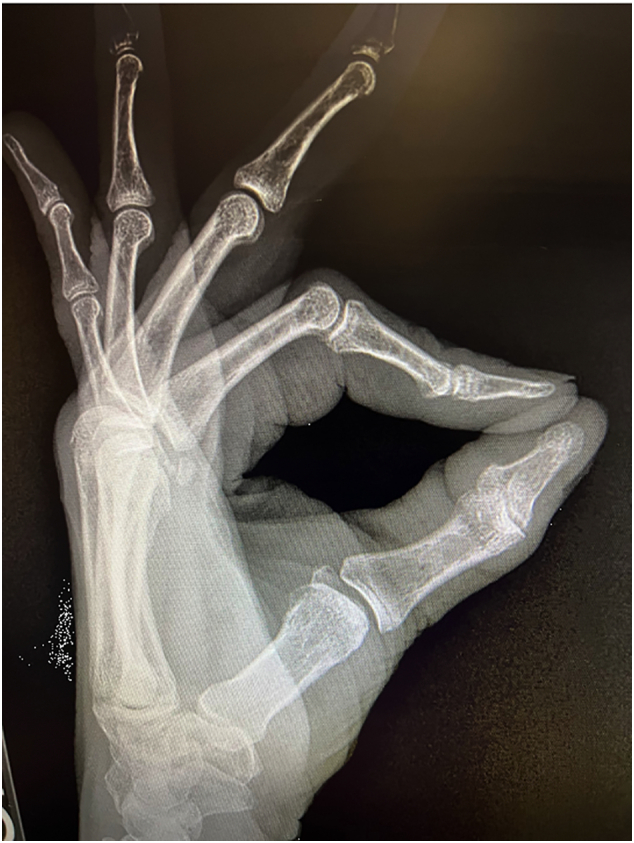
Radiographic image of hand.
